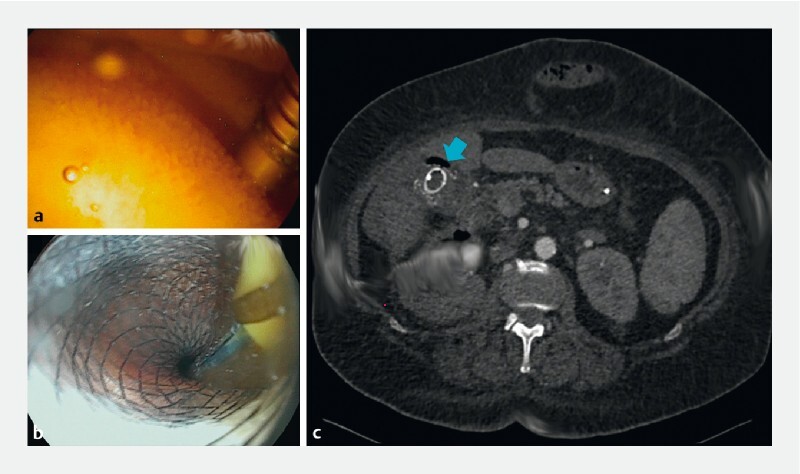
Endoscopic ultrasound-guided lumen-apposing metal stent (20 mm × 16 mm) placement for left kidney drainage through the gallbladder, followed by nasogastric tube placement.
